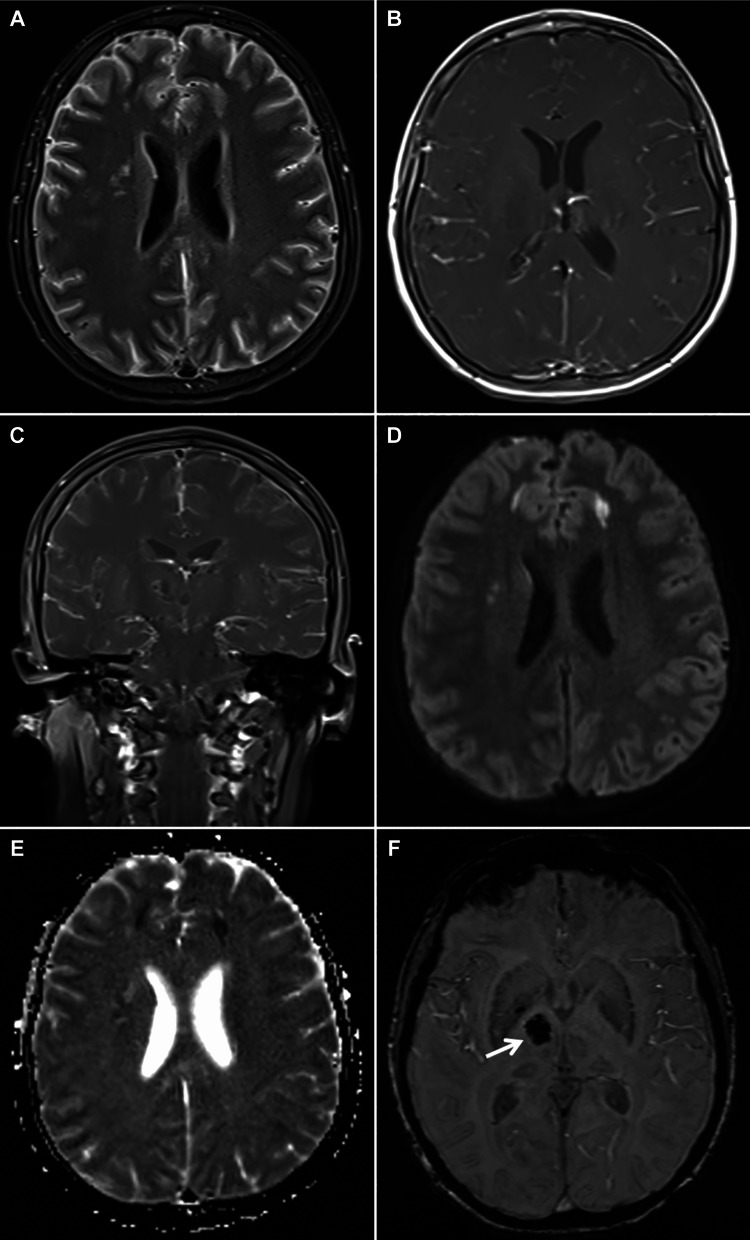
Brain MRI with gadolinium (A) Axial Fluid attenuated inversion recovery (FLAIR) image showing leptomeningeal enhancement.
